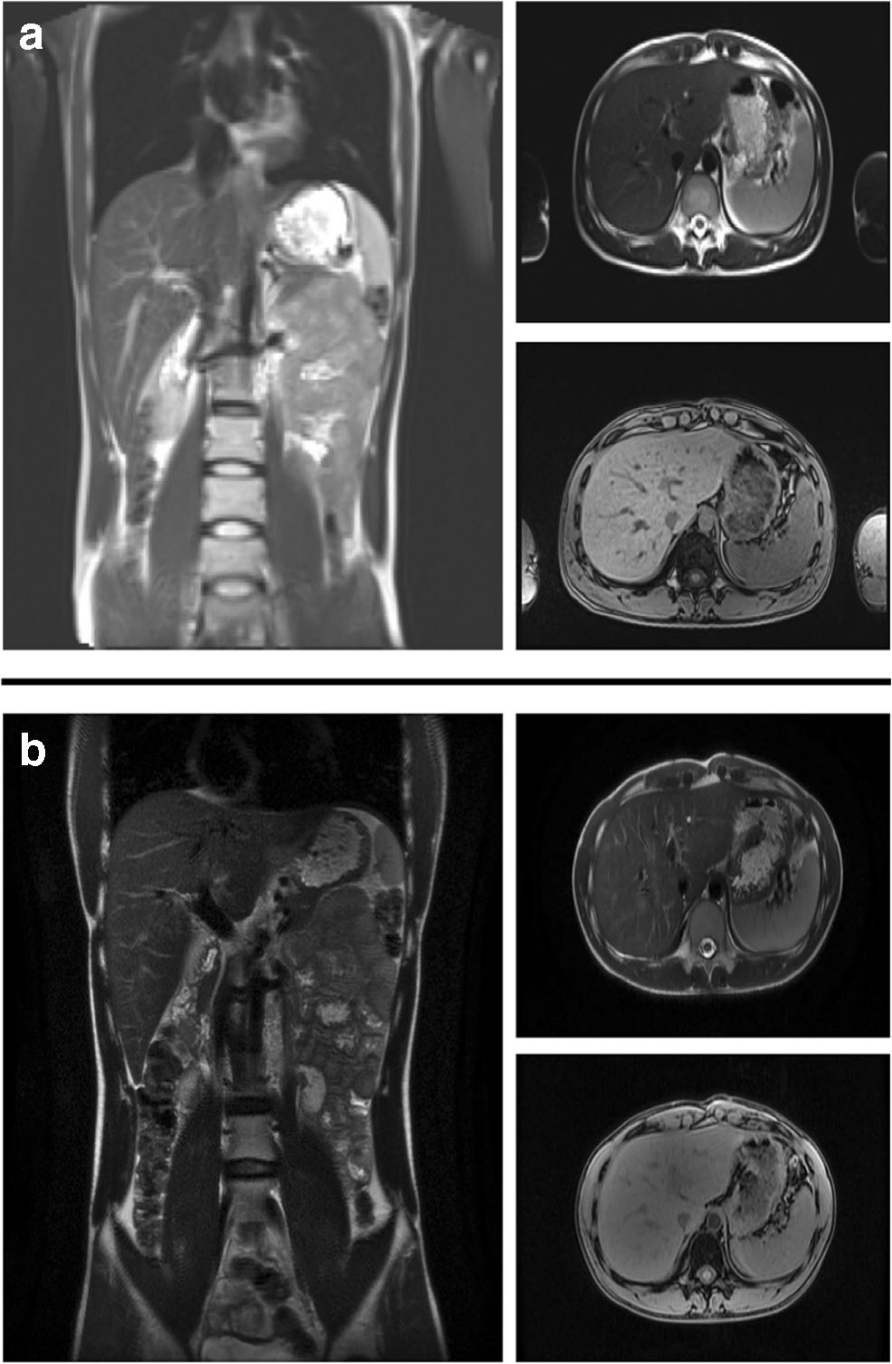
Hepatic MRI for patient 3 in a 2015 and b 2018 (unpublished data)
